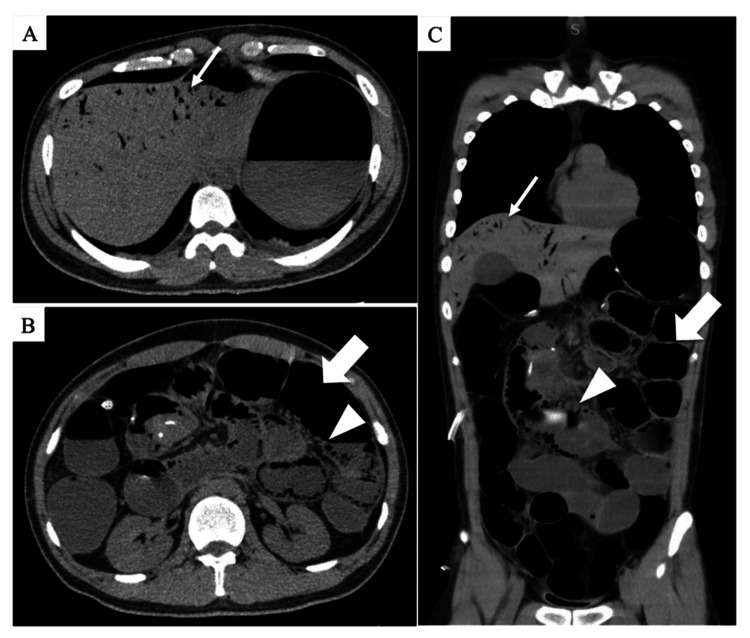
Abdominal CT scan obtained prior to the second surgery. Axial CT scan images showing bowel distension (arrow) (A, C) and emphysema of the intestinal wall (arrowhead) and portal vein (narrow arrow) (B, C). These findings suggest a significant increase in intestinal pressure and mucosal injury, strongly indicating the possibility of intestinal necrosis and severe ischemia. CT: computed tomography
